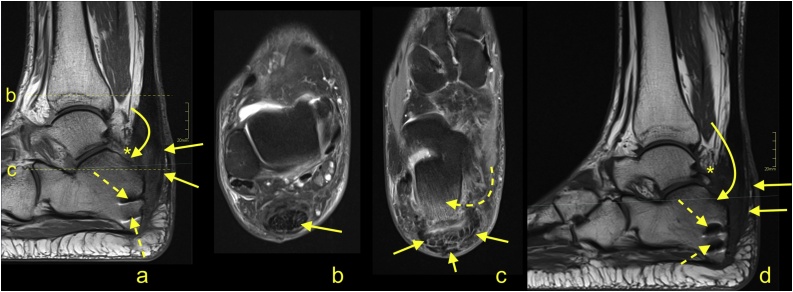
Postoperative infection of the Achilles tendon insertion after surgery for Haglund's deformity. Surgery was performed 5 weeks ago. The wound is not healing, and now, there is redness and swelling of the Achilles tendon insertion. Alteration of the structure, loss of fibrillar structure of the tendon with edema, and reactive changes in the subcutaneous tissue are visible (straight arrow); however, it is challenging to distinguish postoperative changes from infections based only on MRI (a-d). The presence of abscess was ruled out after contrast injection (not seen in the figure). A postoperative defect in the calcaneus (curved arrow) and adhesions with alteration in Kager's fat pad are visible. Diffuse bone marrow edema in the calcaneus is present. Screws in the calcaneus (dashed arrow). a- PD-weighted; b and c - PD-weighted with fat suppression; d - T1-weighted.
